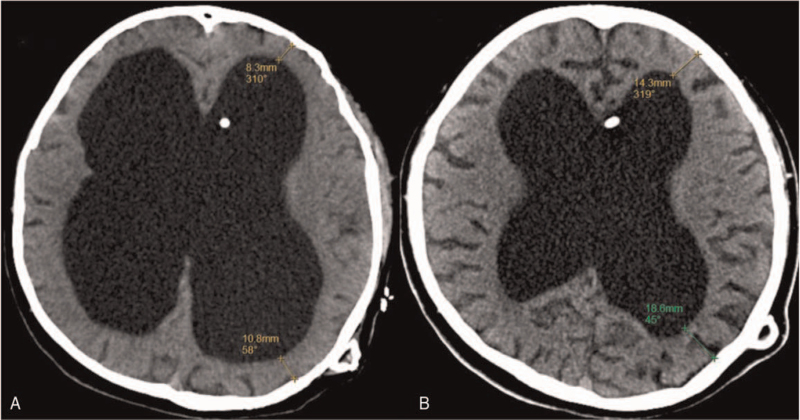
Preoperative and postoperative (2 years of follow-up) CT of the patient. CT demonstrated that the shortest distance from the anterior horn of the lateral ventricle to the skull was 8.3 mm and the shortest distance from the posterior horn of the lateral ventricle to the skull was 10.8 mm (A). CT demonstrated that the shortest distance from the anterior horn of the lateral ventricle to the skull was 14.3 mm and the shortest distance from the posterior horn of the lateral ventricle to the skull was 18.6 mm. The results showed that the ventricle size and ICP decreased, brain compliance increased and brain tissue improved. CT = computed tomography, ICP = intracranial pressure.
